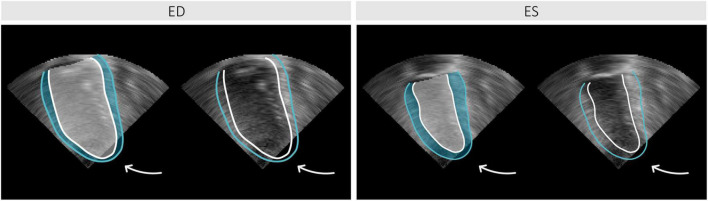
Example of an annotated 3D echocardiography (3DE) image sliced longitudinally at end-diastole (ED) and end-systole (ES), showing portions of labeled regions and corresponding contours for the left ventricular cavity (white) and myocardium (blue) falling outside the acquired 3DE pyramidal volume (as indicated by the arrows).
